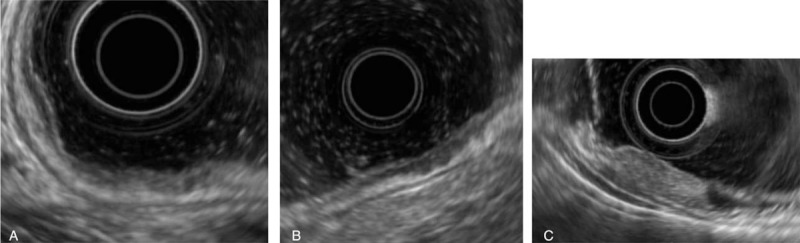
Endoscopic ultrasound images of ulcerative early gastric cancers. (A) Endoscopic ultrasonography (EUS)-mucosa, a hypoechoic tumor disrupted the muscularis mucosa, but with the hyperechoic layer 3 (submucosa) intact. (B) EUS-submucosa, a hypoechoic tumor invading the submucosa. (C) EUS-advanced lesion, a hypoechoic tumor invading the muscularis propria.
